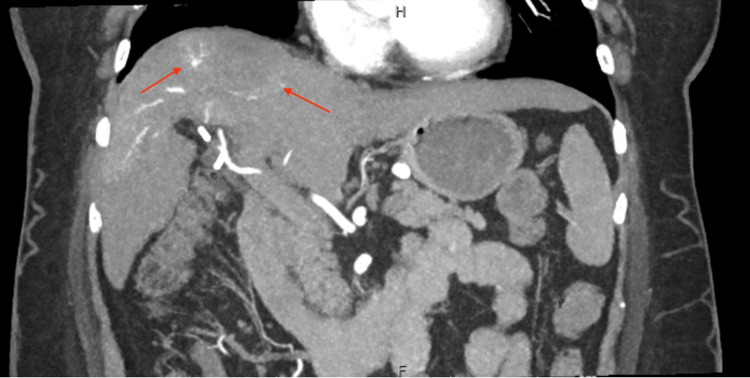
Computerized tomography (CT) scan showing a mass measuring 3.8x4.1x3.2 cm with slight peripheral nodular enhancement during the arterial phase.
